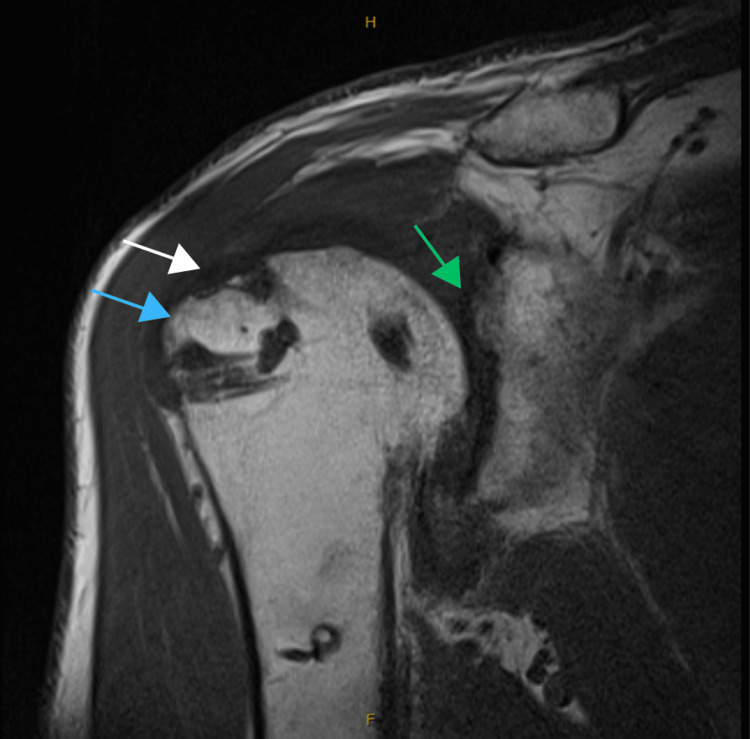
MRI of the left shoulder Coronal T1 MRI of the left shoulder with the white arrow indicating a high-grade partial thickness tear of the supraspinatus tendon, superimposed upon underlying tendinopathy and mild muscle atrophy. The blue arrow is displaying posterior subluxation of the greater tuberosity with respect to the glenoid. The green arrow indicates a degenerative type I and possibly type II superior labrum anterior to posterior tears.
